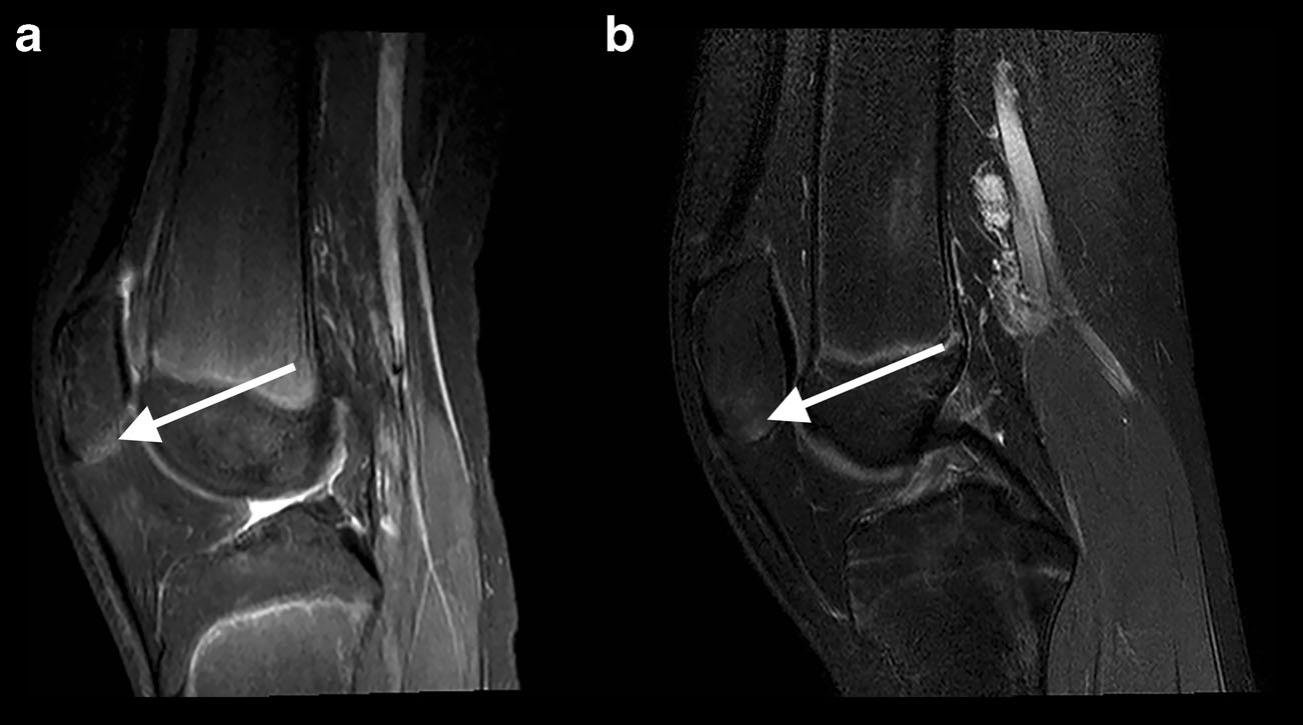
Bone marrow changes in two different healthy children (arrow). Sagittal T2-weighted fat saturated images
